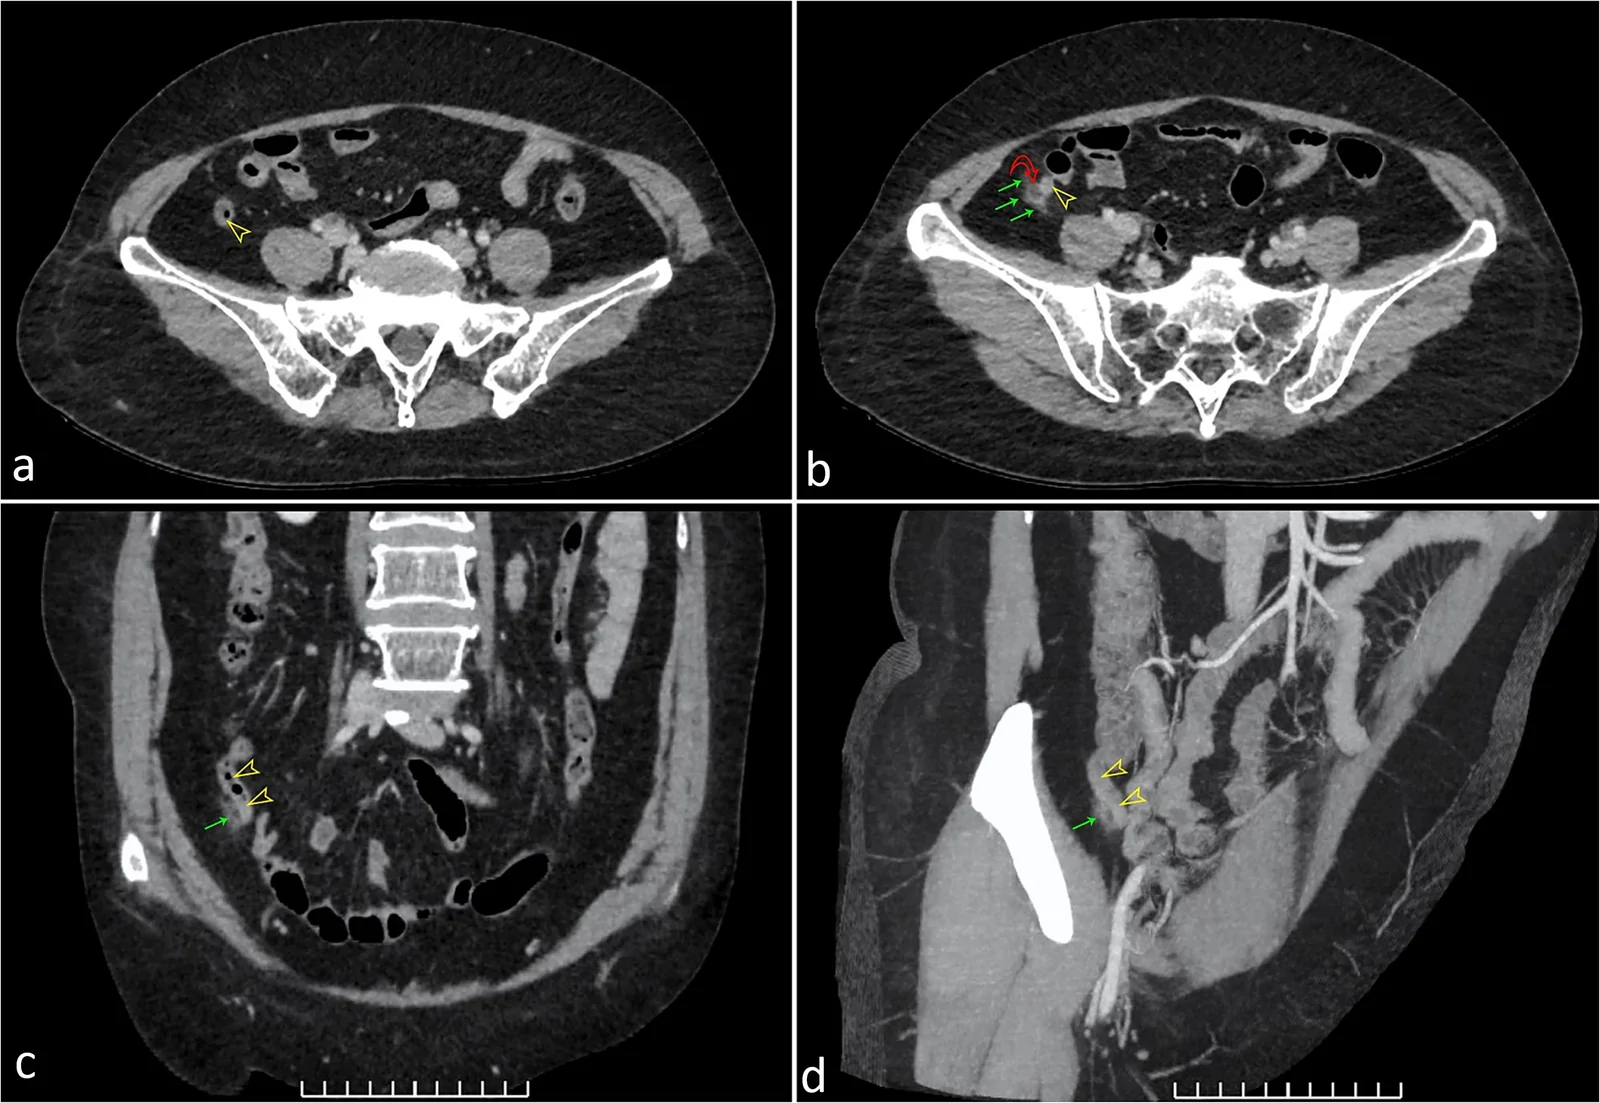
Contrast-enhanced CT images in (a, b) axial, (c) coronal, and (d) oblique coronal planes with MIP reconstruction show a mildly enlarged appendix (arrowheads), containing fluid and gas. Adjacent to the distal portion of the appendiceal body, there is a round, fat-density structure (curved arrow) with mild surrounding fat stranding (small arrows).
